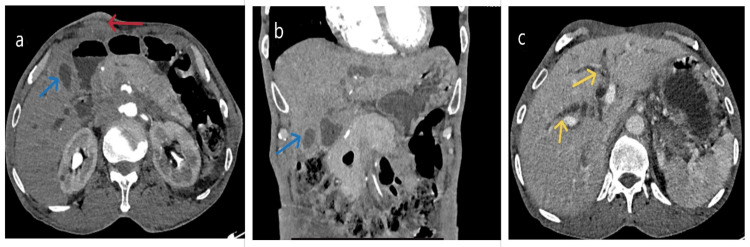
CECT abdomen axial and coronal sections (a-c). Ill-defined, circumferential thickening of the gallbladder's fundus, body, and neck (blue arrow), abutting liver segments IVB, V, and the hepatic flexure of the colon, with indistinct fat planes and associated intrahepatic biliary radicle dilatation (yellow arrows). An enhancing lesion in the skin and subcutaneous tissue of the right hypochondrium (red arrow) which is non-contiguous with the GB thickening. CECT: contrast-enhanced computed tomography; GB: gallbladder
